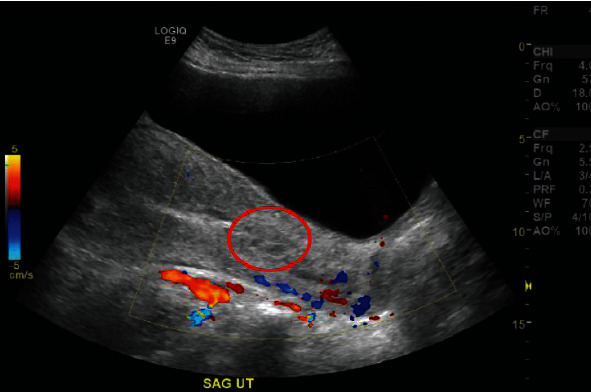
Transvaginal ultrasound of the uterus. Sagittal view of the uterus demonstrated complex echogenic area in the lower uterine segment without Doppler flow, consistent with a blood clot (encircled in red) measuring approximately 2.9 cm in diameter.
